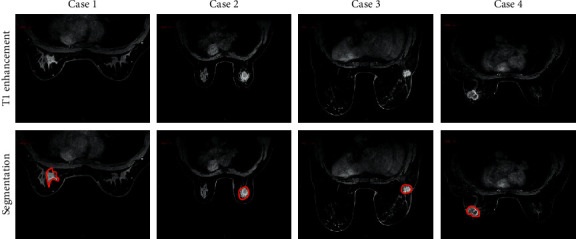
MRI images of breast cancer distant metastasis and the result of algorithm segmentation of the MRI image target area. Case 1 was 58 years old, case 2 was 35 years old, case 3 was 49 years old, and case 4 was 62 years old. The red box marked the specific observation positions.
